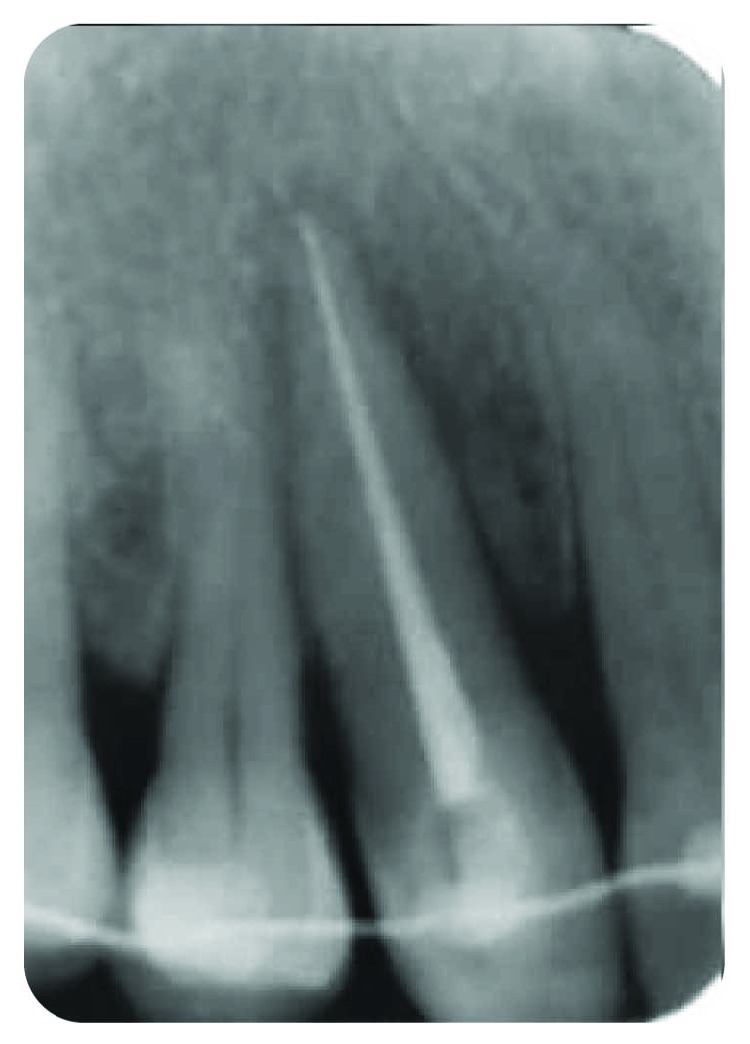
39 months' recall radiograph showing a bone reorganization.
